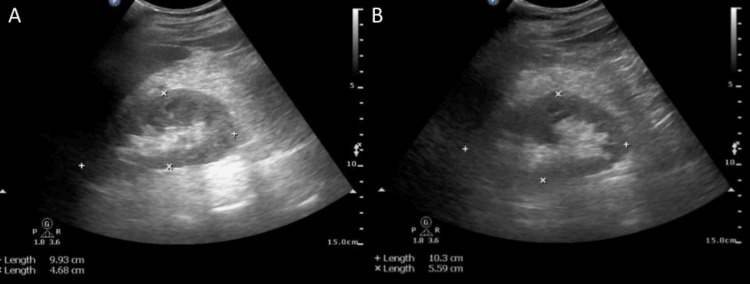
Ultrasonography of (A) right and (B) left kidneys, normal size with increased parenchymal echogenicity
